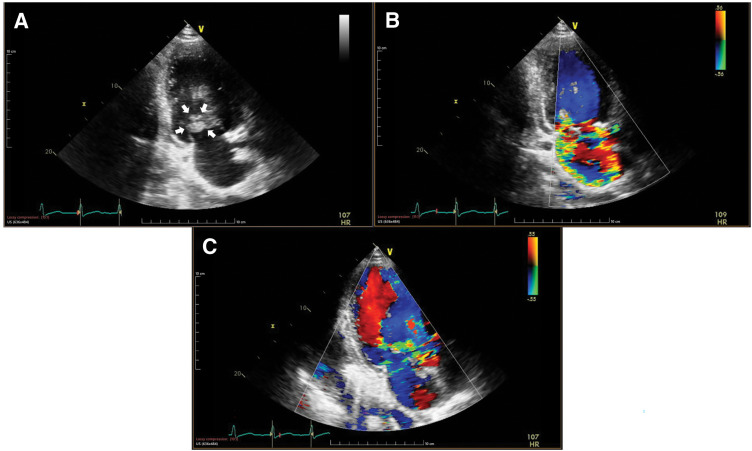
Preoperative TTE. TTE revealed marked thickening of the anterior mitral leaflet with a large mass attached to it, suggestive of either a large vegetation or aneurysmal leaflet deformation (A, arrows), along with severe MR (B) and severe AR (C). AR, aortic regurgitation; MR, mitral regurgitation; TTE, transthoracic echocardiography
